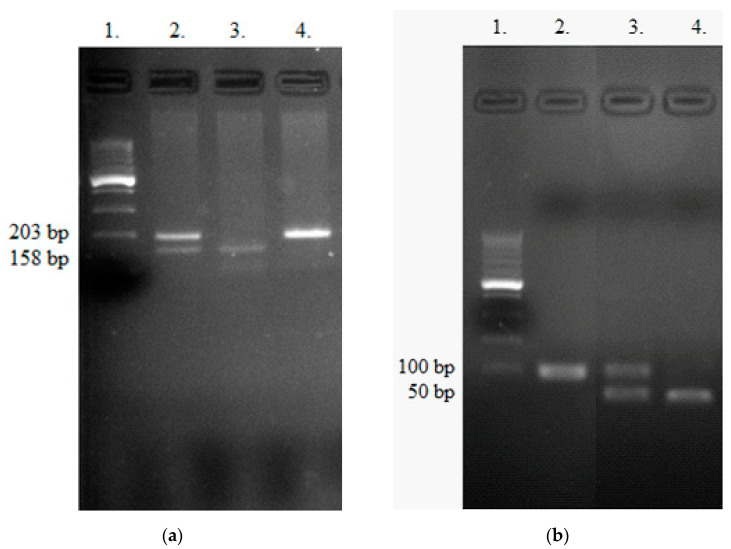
Detection of 6746T>C *NOTCH3* (a) and -8C>G *PSMA6* (b) polymorphisms. (a) Lane 1—Ladder (100bp); Lane 4 contains TT genotype; Lane 2 contains TC heterozygote; Lane 3 contains CC genotype. (b) Lane 1—Ladder (100bp); Lane 2 shows CC genotype; Lane 3—CG heterozygote, Lane 4—GG homozygote.
